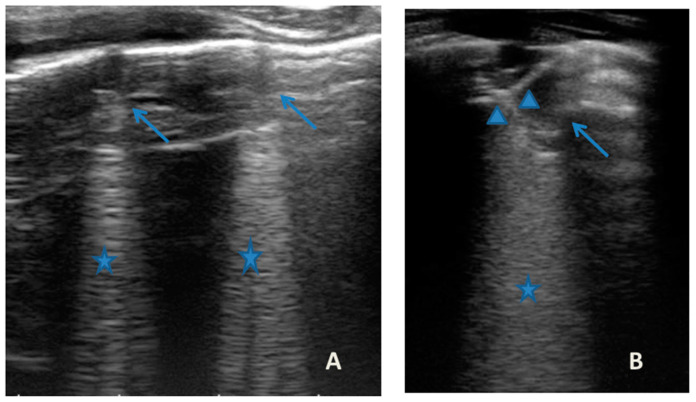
Grayscale lung ultrasound examination shows: (A): sub-centimeter consolidations less than 0.5 cm in size (arrows), associated with confluent artifacts (asterisks) in the left lateral mid-apical area; (B): a small consolidation of 0.5–1 cm (arrows), with elements of dynamic air bronchogram (arrowhead) (as per suspected inflammation) [10,14,15], associated with a circumscribed area of “white lung” (asterisks) in the right middle apical area; (A,B): irregularity of the pleural line. Picture compatible with acute inflammation of the small airways of viral origin [14,15].
